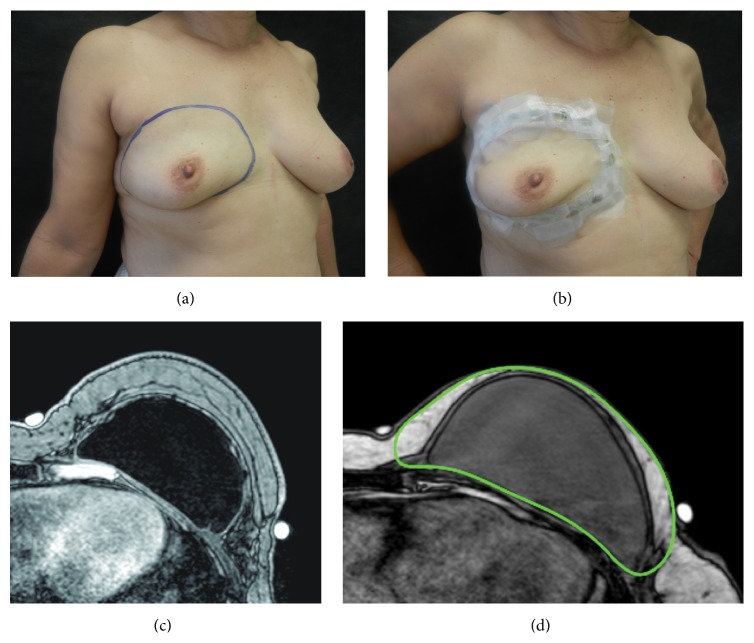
Method for MRI-based breast volume measurement. (a) Breast boundaries demarcated, (b) external markers applied, (c) axial sequence showing the breast medial and lateral limits, and (d) a selected region of interest (ROI) with the OsiriX software.
